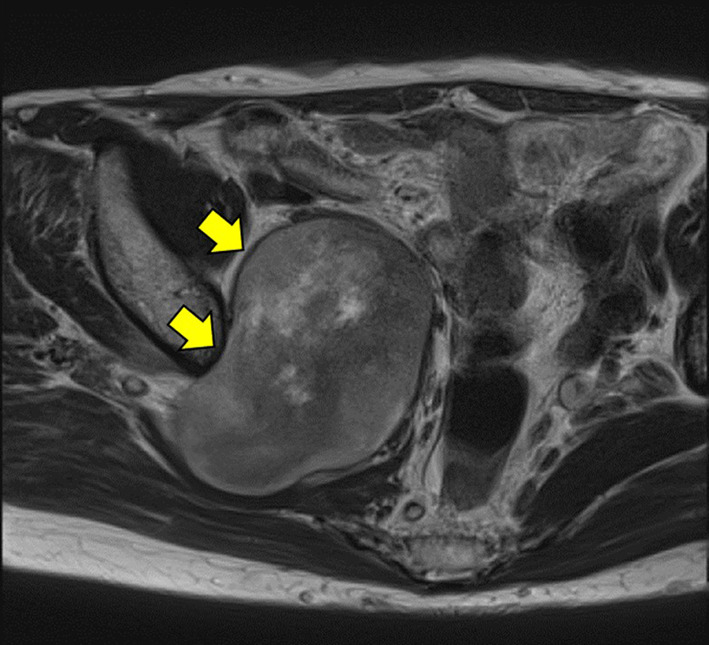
Pelvic MRI. Pelvic MRI shows a heterogeneous high-signal mass on T2-weighted imaging
